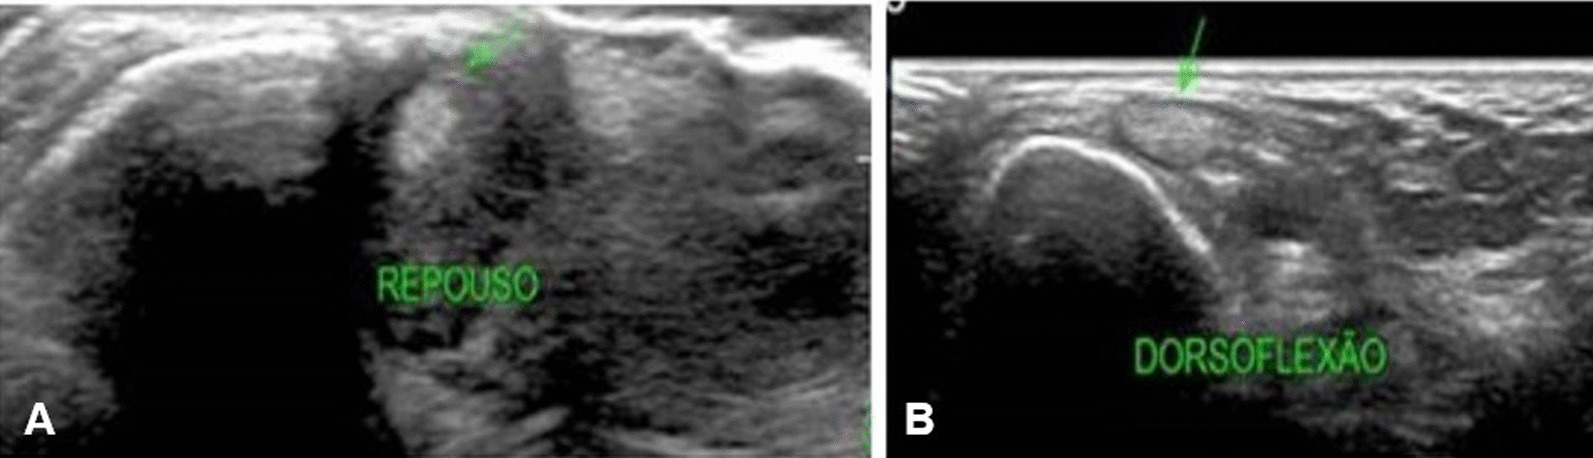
A Ultrasound of the left ankle, displaying reduced peroneus brevis tendon, in resting position (green arrow). B Subluxated peroneus brevis tendon during dorsiflexion (green arrow)
